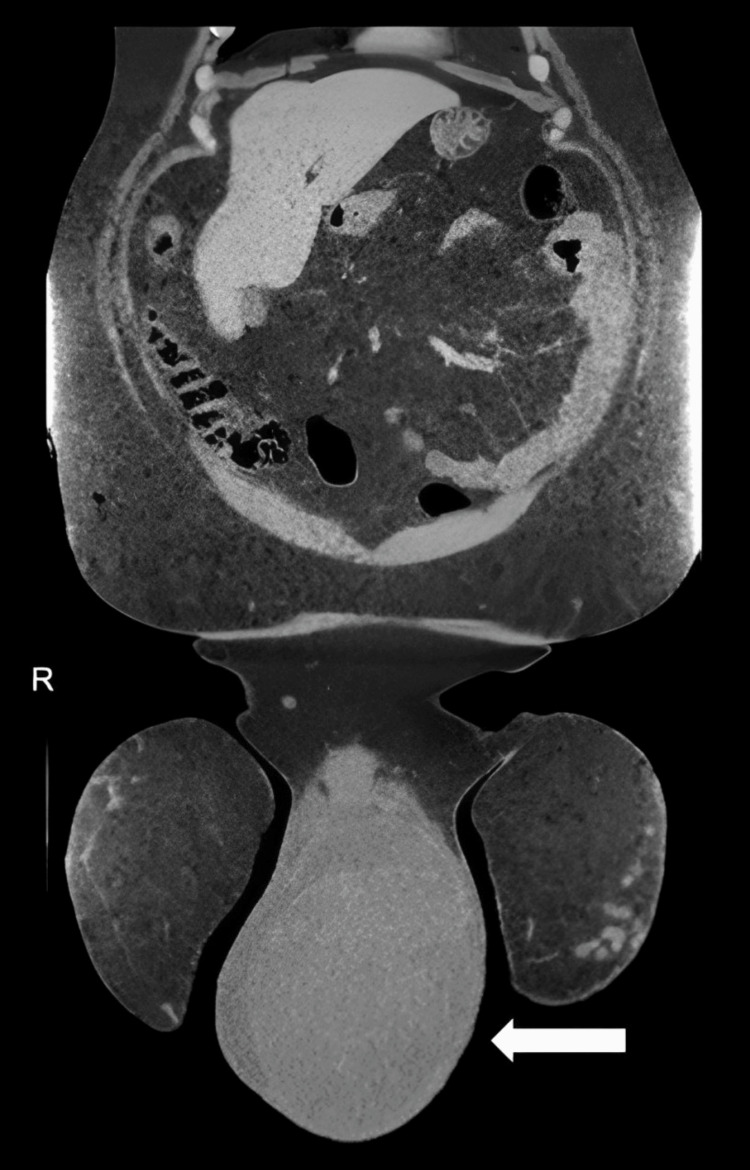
Coronal CT image showing the testicular mass
